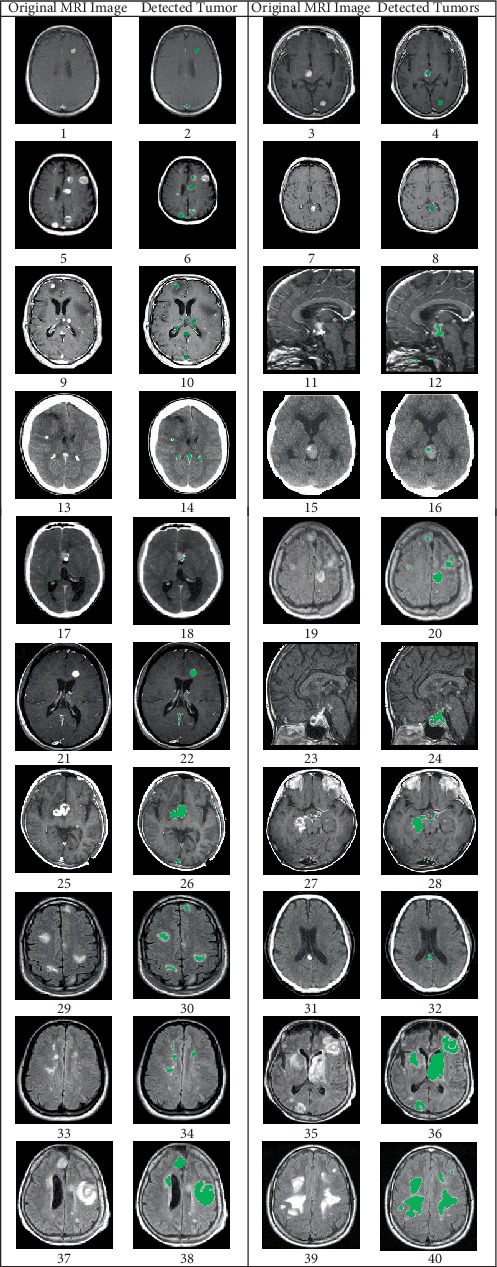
MRI brain images that contain tumors with different sizes and intensity levels and the detected tumors in each image.
